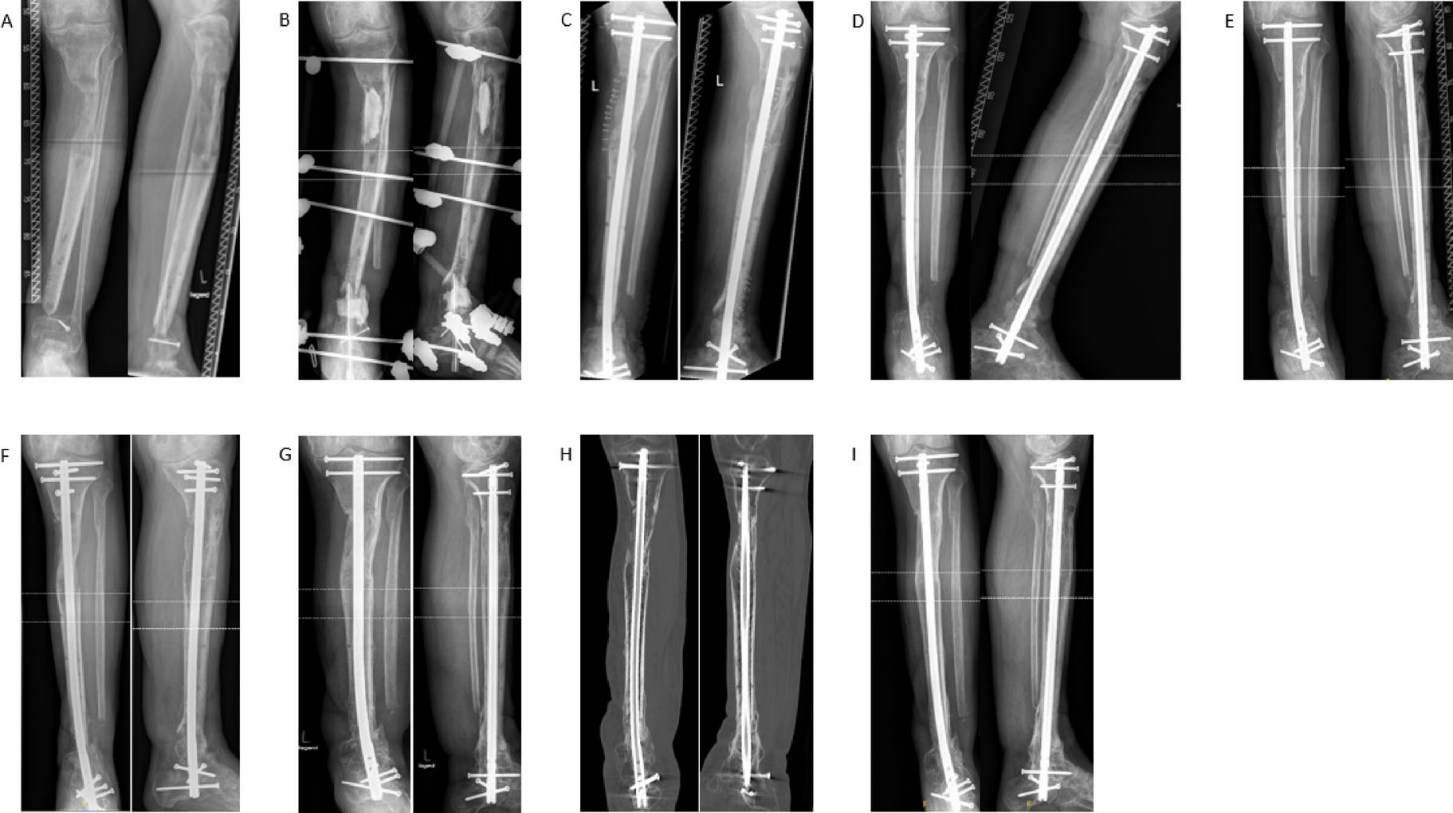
Example of a patient with a distal tibia non-union undergoing a two-step procedure receiving a ETN protect (A) initial X-rays presenting the first time in our department (B) X-rays two days after the first step of the Masquelet therapy with debridement of the non-union and resection of the sclerotic avascular bone, the bone defect was filled with a PMMA spacer, stabilization was achieved using an external fixator (C) X-ray two days postoperative after Step 2 of the induced membrane technique with change to an ETN protect, removal of the spacer and augmentation of the bony defect with autologous bone in RIA technique from the ipsilateral femur in combination with VITOSS and BMP-7 (D) X-ray image 6 weeks postoperative (E) X-ray image 12 weeks postoperative (F) X-ray image 6 months postoperative (G) X-ray image 1 year postoperative (H) CT scan 1 year postoperative with complete bony consolidation (I) X-ray image 2 years postoperative with ongoing remodelling.
